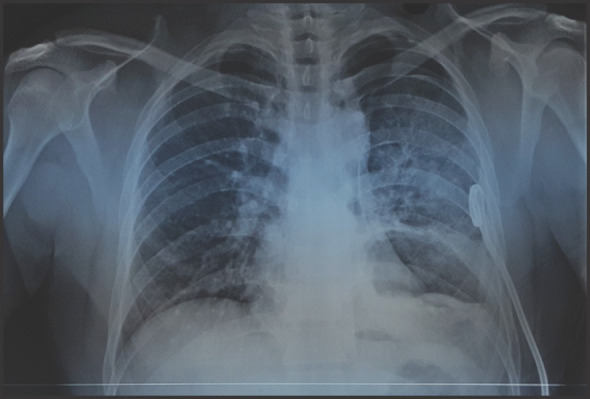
Chest x-ray after pigtail insertion showing lung expansion.
